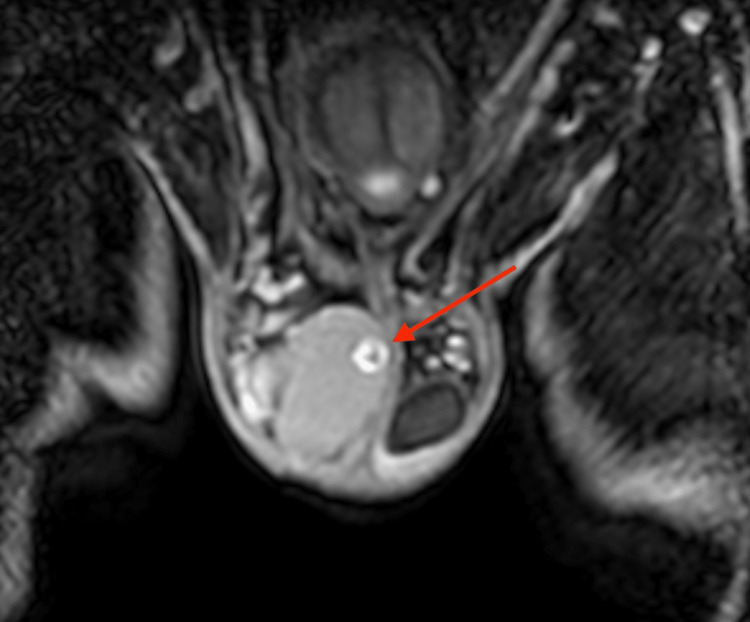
Contrast-enhanced, fat-suppressed, T1-weighted coronal MRI of the pelvis Image of the scrotum and right testicle and coronary view of both thighs showing a contrast-enhanced tumor in the right testicle (marked with the arrow)
